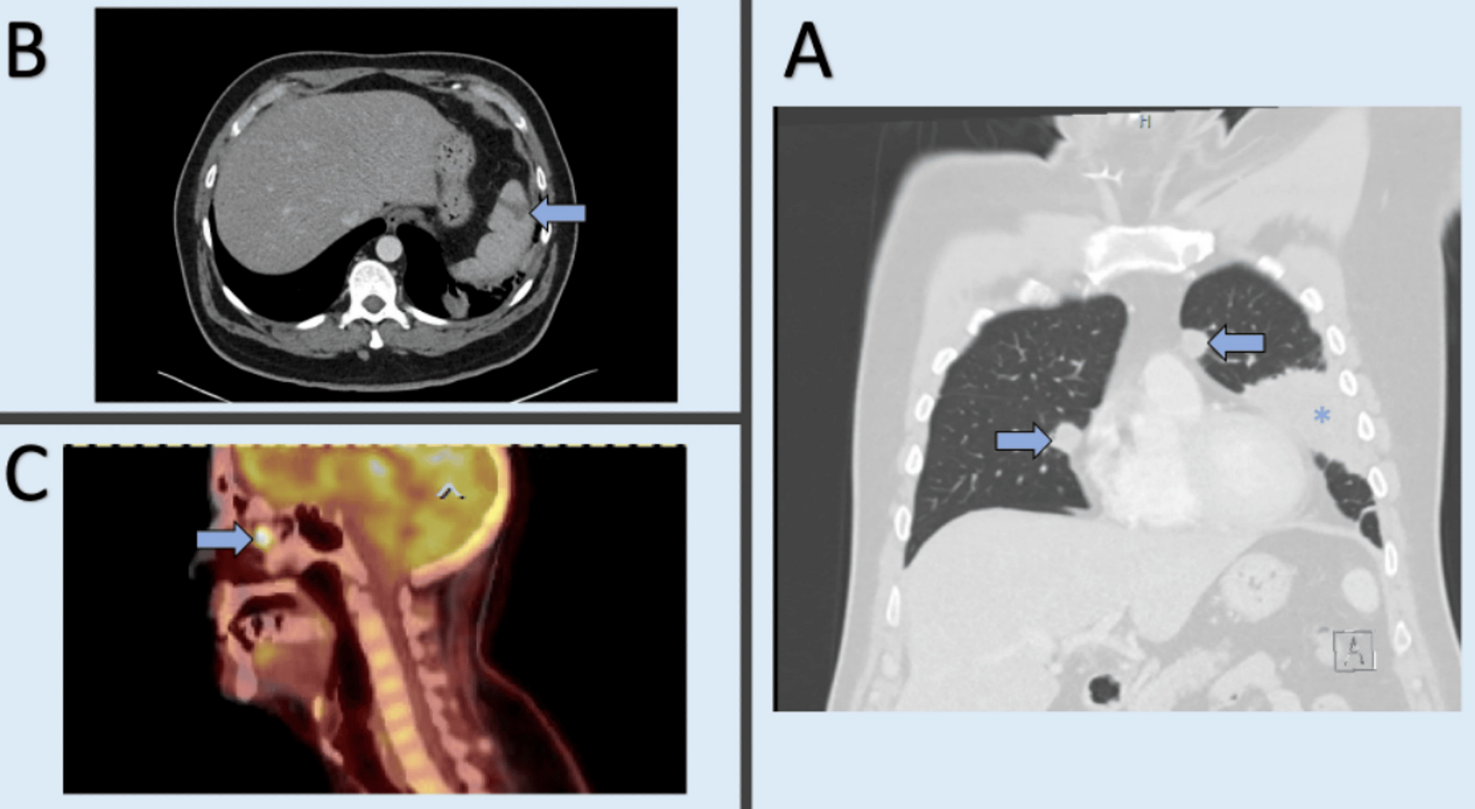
Imaging findings (A) CT chest showing a nodular cavitary process in the lung fields (arrows) and lingular consolidation (asterisk); (B) wedge-shaped hypo-perfusion within the spleen, consistent with splenic infarction (arrow); (C) PET scan showing abnormal increased activity in the nasal area (arrow).
